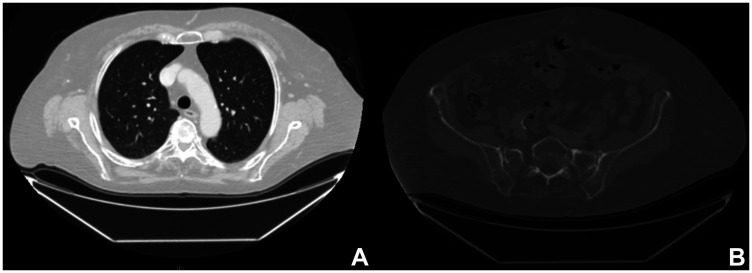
Radiological images after combined targeted therapy. Computed tomography one year after BRAF/MEK-i therapy, showing a complete response of the disease with regression of the lung (A) and bone metastasis (B).
